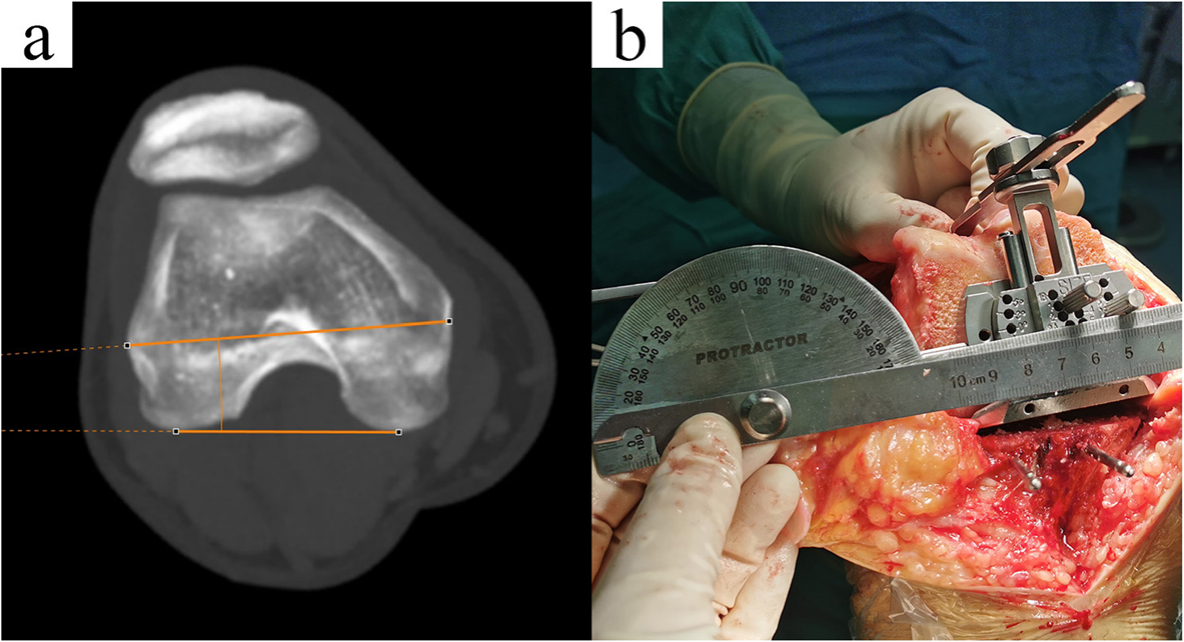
Transepicondylar axis angles (TEAAs) were measured by preoperative superimposed CT (a) and a protractor in the operating room (b). a Determination of the recess of the medial epicondyle, the prominence of the lateral epicondyle, and the highest point of the medial and lateral posterior condyles by the MIP approach of CT. The TEAA value was automatically obtained by the in-house program. b Intraoperative measurement required electrocautery to mark the position of the medial and lateral epicondyles on the distal femoral resection surface. The arms of the protractor were parallel to the posterior pedals of instruments and the electrocautery marks
